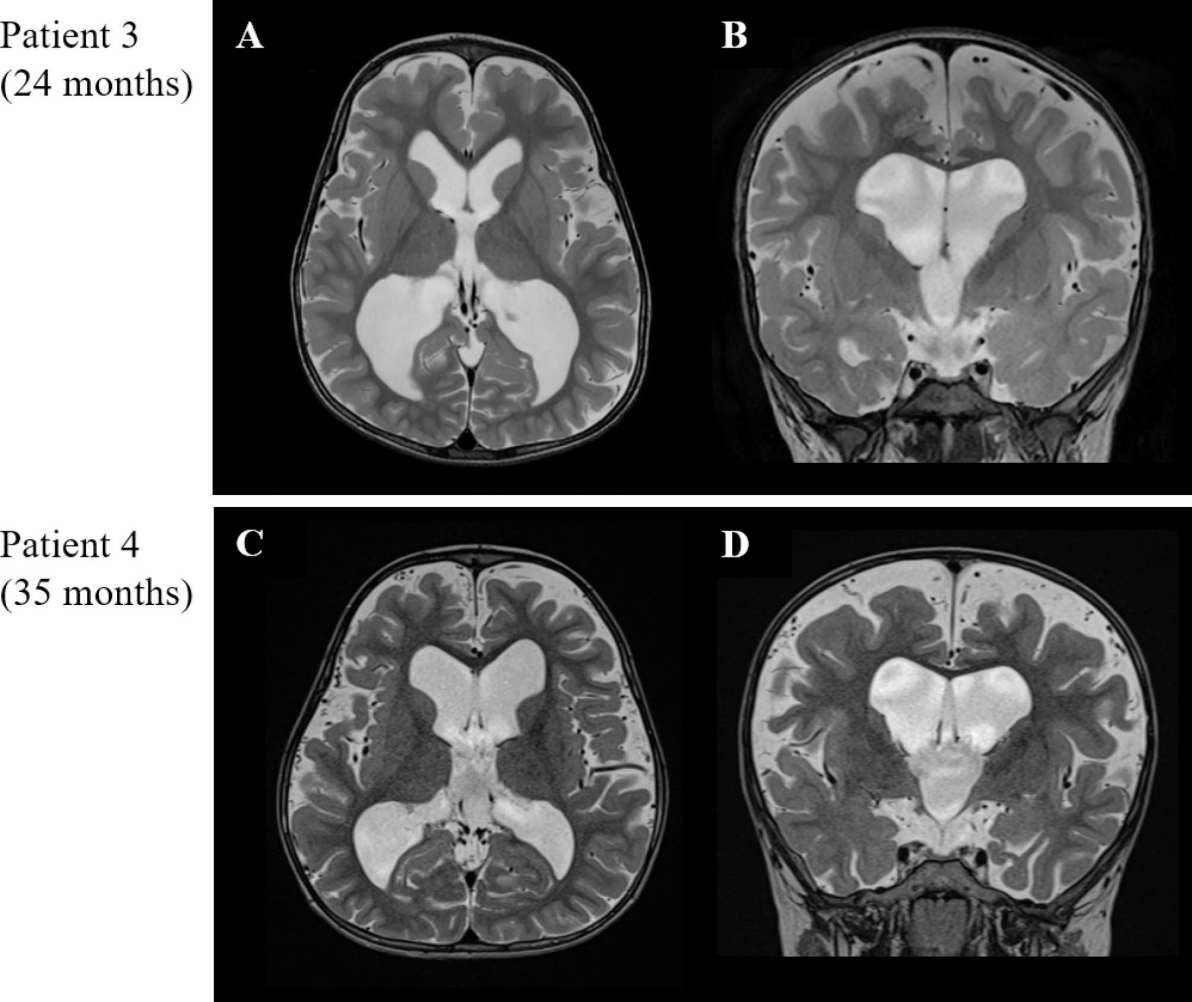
Brain magnetic resonance imaging (MRI) was taken in two achondroplasia patients who didn’t undergo ventriculoperitoneal shunt. Each was performed at 24 months (patient 3, A, B) and 35 months (patient 4, C, D) of age. Both patients showed mild to moderate dilatation of lateral and third ventricles with excessive cerebrospinal fluid volume in the cortical subarachnoid space on T2-axial (A, C) and T2-coronal (B, D) images. (A, B) Frontal horn width = 57.58 mm, Evan’s ratio = 0.36 (C, D) Frontal horn width = 67.03 mm, Evan’s ratio = 0.39
